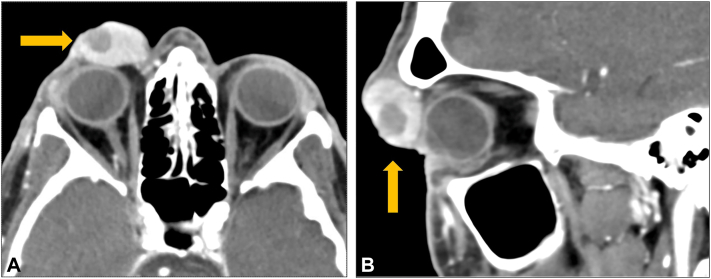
Preoperative imaging of the orbit. Coronal (A) and sagittal (B) images of a computed tomography scan of the orbit and the adjacent MCC (marked by *arrows*) that did not invade surrounding structures. A small area of central necrosis vs postbiopsy fluid was present in the tumor. *MCC*, Merkel cell carcinoma.
